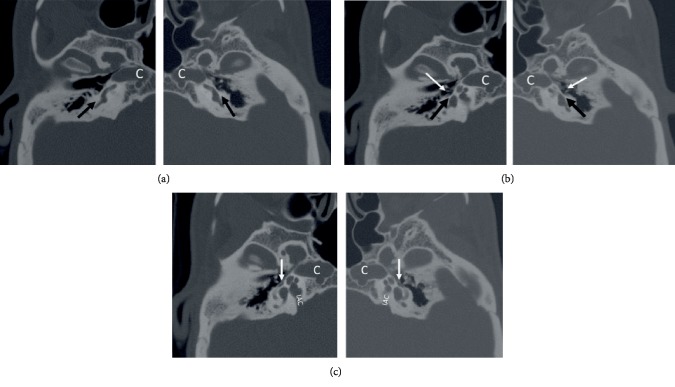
(a) High resolution axial CT Temporal bone images at the level of round window (a), stapes and oval window (b), and tympanic facial nerve (c). Absence of bilateral round ((a), dark arrows) and oval windows ((b), dark arrows) with tympanic facial nerve covering the site of oval window (C, arrows). Stapedial anomalies present with aplastic crura on the right and nearly intact left stapes except hypoplastic anterior crus (white arrows, (b)). Note skull base dysmorphism with abnormal orientation of internal auditory canal (IAC) and kissing carotid canals (marked as C in Figures 3(a)–3(c)).
